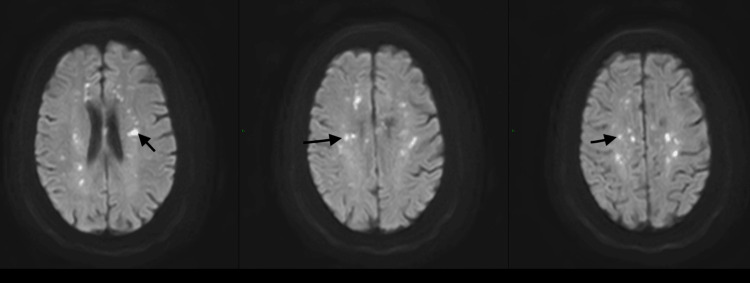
MRI showing extensive bilateral subacute cerebral infarcts (arrows).
